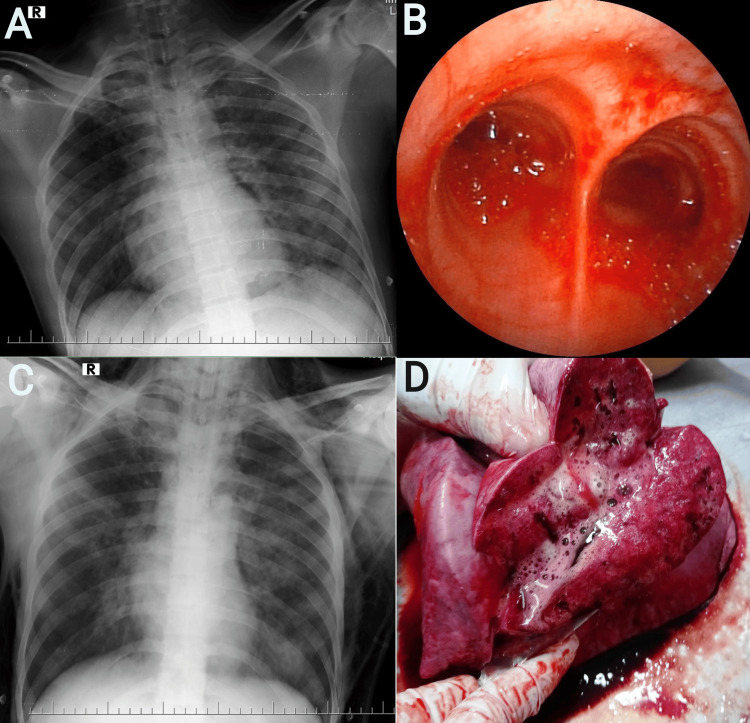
A) Chest radiograph revealed bilateral diffuse infiltrates (left>right) with hilar prominence. B) Flexible bronchoscopy revealed frothy blood flooding until the bilateral mainstem bronchi with airway congestion. C) Chest radiograph revealed bilateral subcutaneous emphysema (left>right) with cephalization of vasculature and persistent pulmonary infiltrates. D) Post-mortem showed frothy secretions from the lungs.
